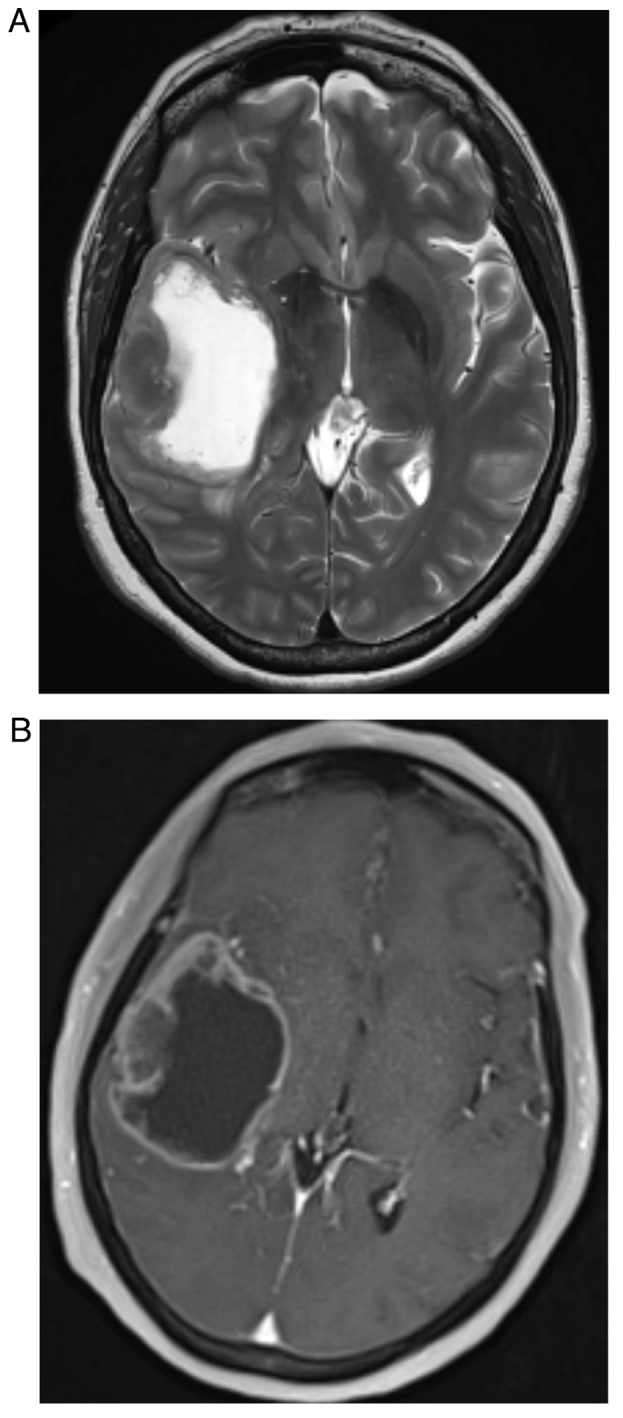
T1 and T2 MR images demonstrating the metastatic tumour. (A) T2 MRI sequence demonstrating high signal intensity within a cystic centre surrounded by thickened heterogenous rim of tumour and mild perilesional change. (B) Post-contrast T1 MRI sequence demonstrating heterogeneously peripheral rim enhancing tumour. MRI, magnetic resonance imaging.
